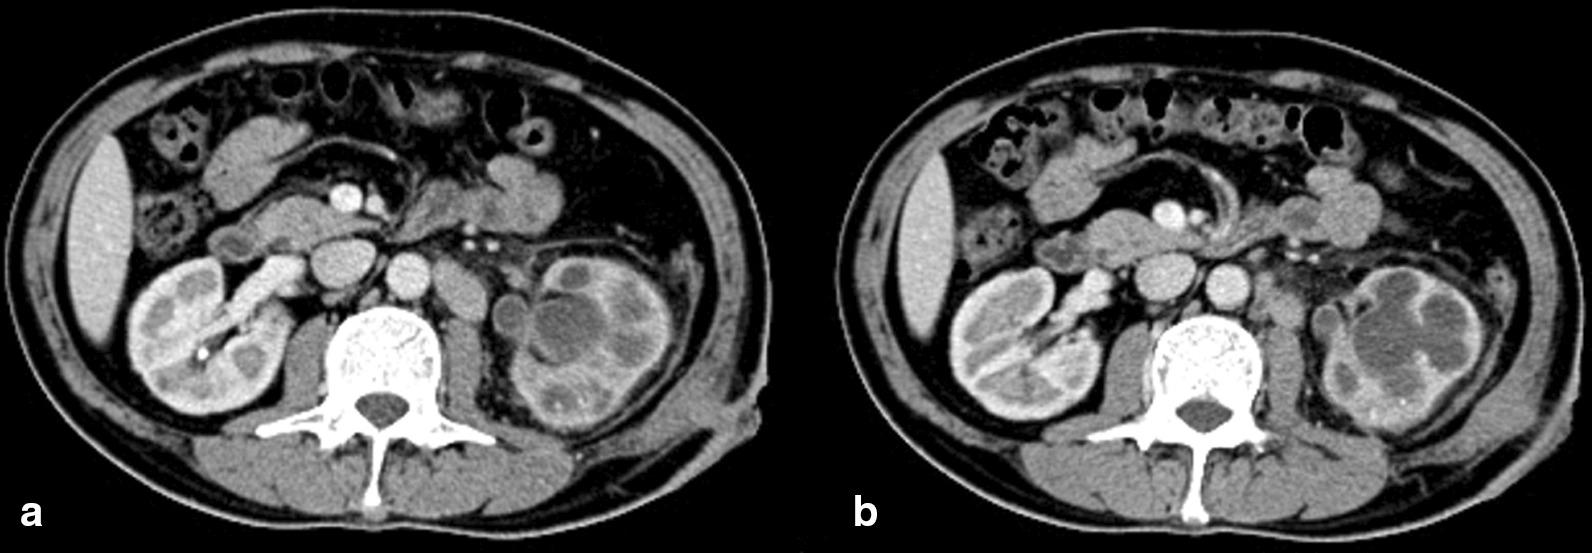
a Case 3 Computed tomography (CT) revealed atrophy of the left renal cortex, stone obstruction, and formation of the left perirenal sinus; b Enhanced CT revealed “bear’s paw” sign in the left kidney
